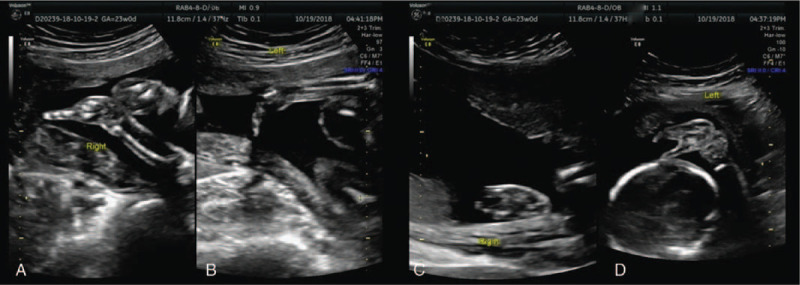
The systemic ultrasound examination of this fetus at the second trimester. It presented with deformed hands and feet. In hands, aplasia of the phalanges and metacarpals was seen, leaving the left hand only with the 2nd finger(B), the right hand only with the 5th finger(A). Both the feet had deep midline cleft, syndactyly, and aplasia of the some digits, absence of phalanges and metacarpals, giving a characteristic lobster-claw appearance (C, D).
